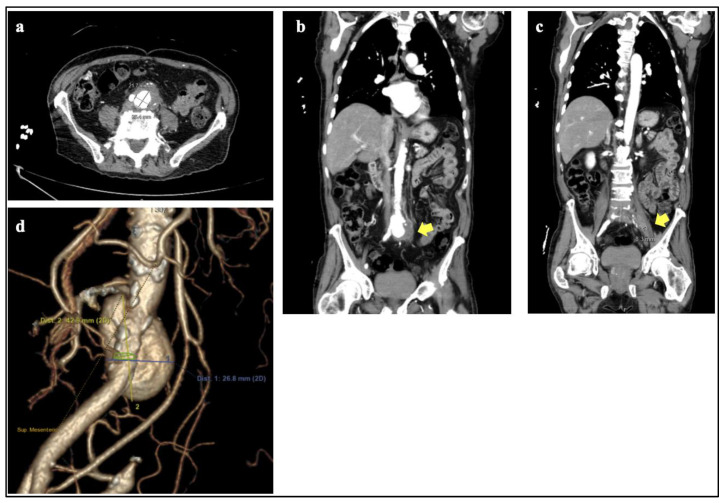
Mycotic aneurysm of abdominal aorta (a) Axial (with measurements) and (b) coronal images of periaortic soft tissue density surrounding saccular aneurysm. (c) Coronal image of periaortic soft tissue density extending into medial left psoas muscle. (d) CTA 3D reconstruction of saccular aneurysm with measurements.
